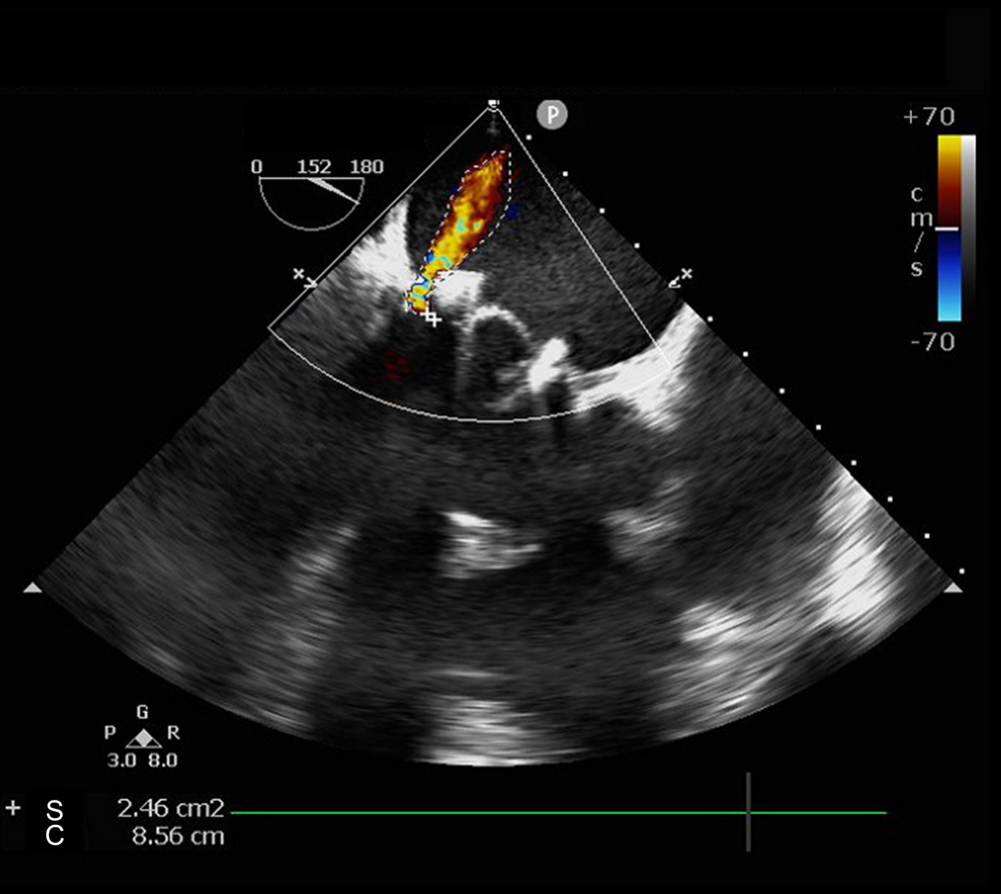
Preoperative transesophageal echocardiography of this patient showing
